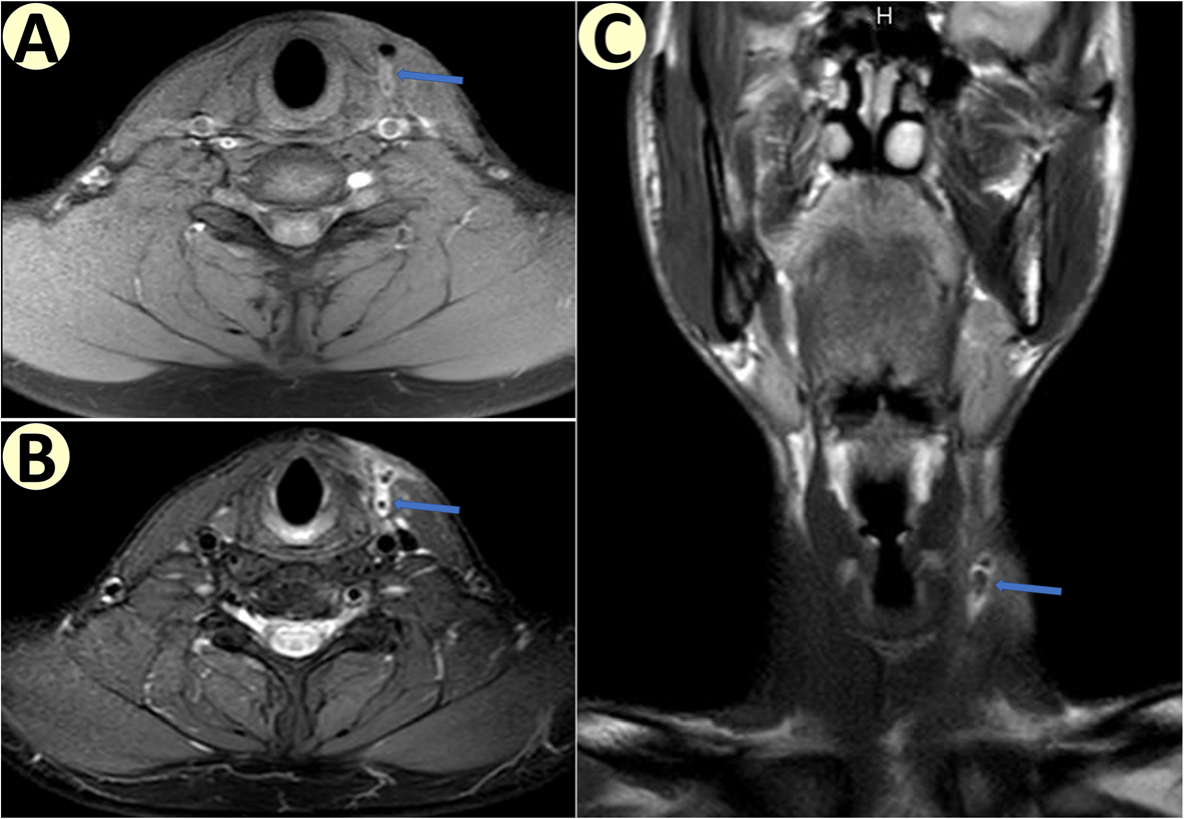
MRI of a left side fourth branchial fistula, as indicated by blue arrow. a T1WI axial image. b T2WI axial image. c T2WI coronal image
